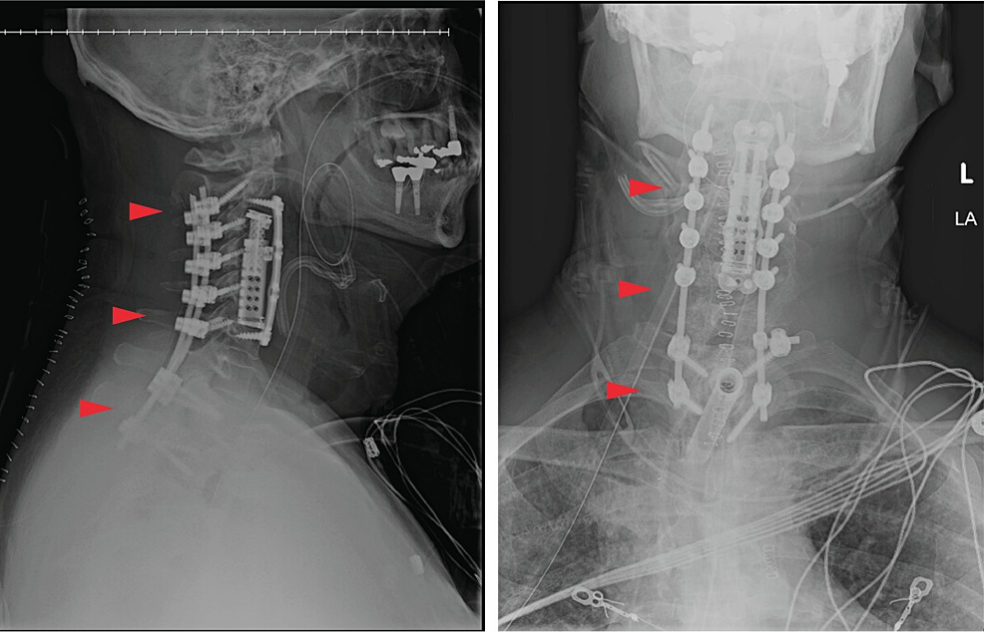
Inpatient postoperative radiographs demonstrating hardware alignment.
